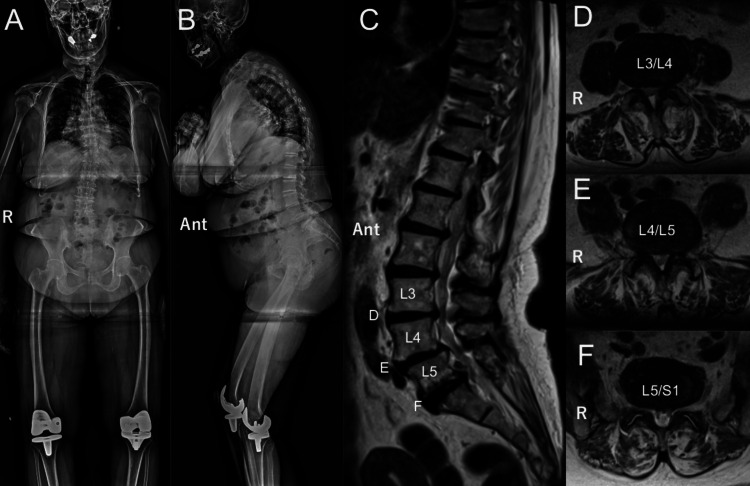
Preoperative radiographic images A: anterior-posterior projection; B: lateral projection; radiographic images display the spinal deformity resulting from lumbar spondylolisthesis; (C) A sagittal magnetic resonance image (MRI) reveals lumbar canal stenosis from L3 to S1 and spondylolisthesis at the L4/5 level; (D-F) The axial MRI images indicate lumbar canal stenosis at distinct levels (d: L3/4, e: L4/5, and f: L5/S1) (Ant: anterior, R: right).
